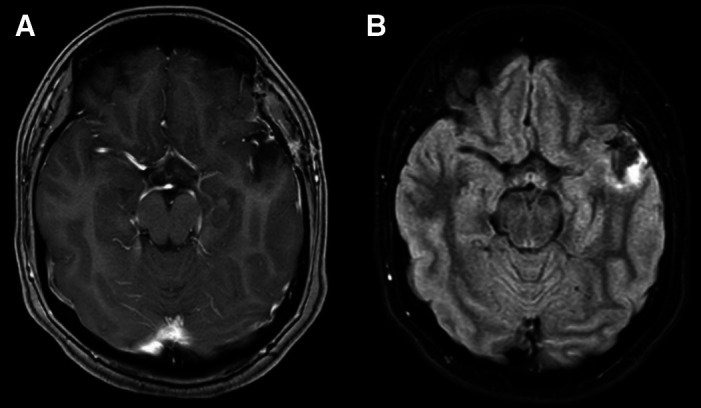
At 6-month follow-up, (A) axial T1-weighted contrast-enhanced magnetic resonance imaging and (B) fluid attenuated inversion recovery sequence showed a noticeable decrease in the lesion of the temporal lobe.
